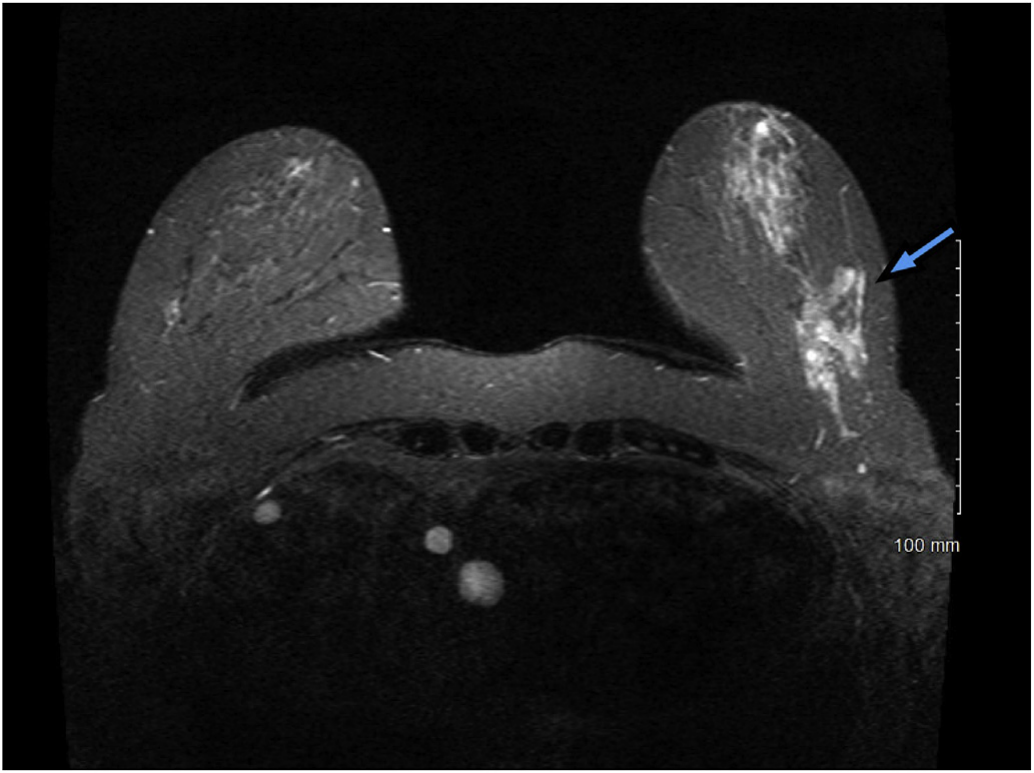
MRI of Left breast demonstrates mild background parenchymal enhancement, Mass enhancement within the lower outer aspect of the left breast which measures 5 cm in AP dimension 3 cm transverse dimension and 2 cm in craniocaudal dimension corresponding to area biopsied.
